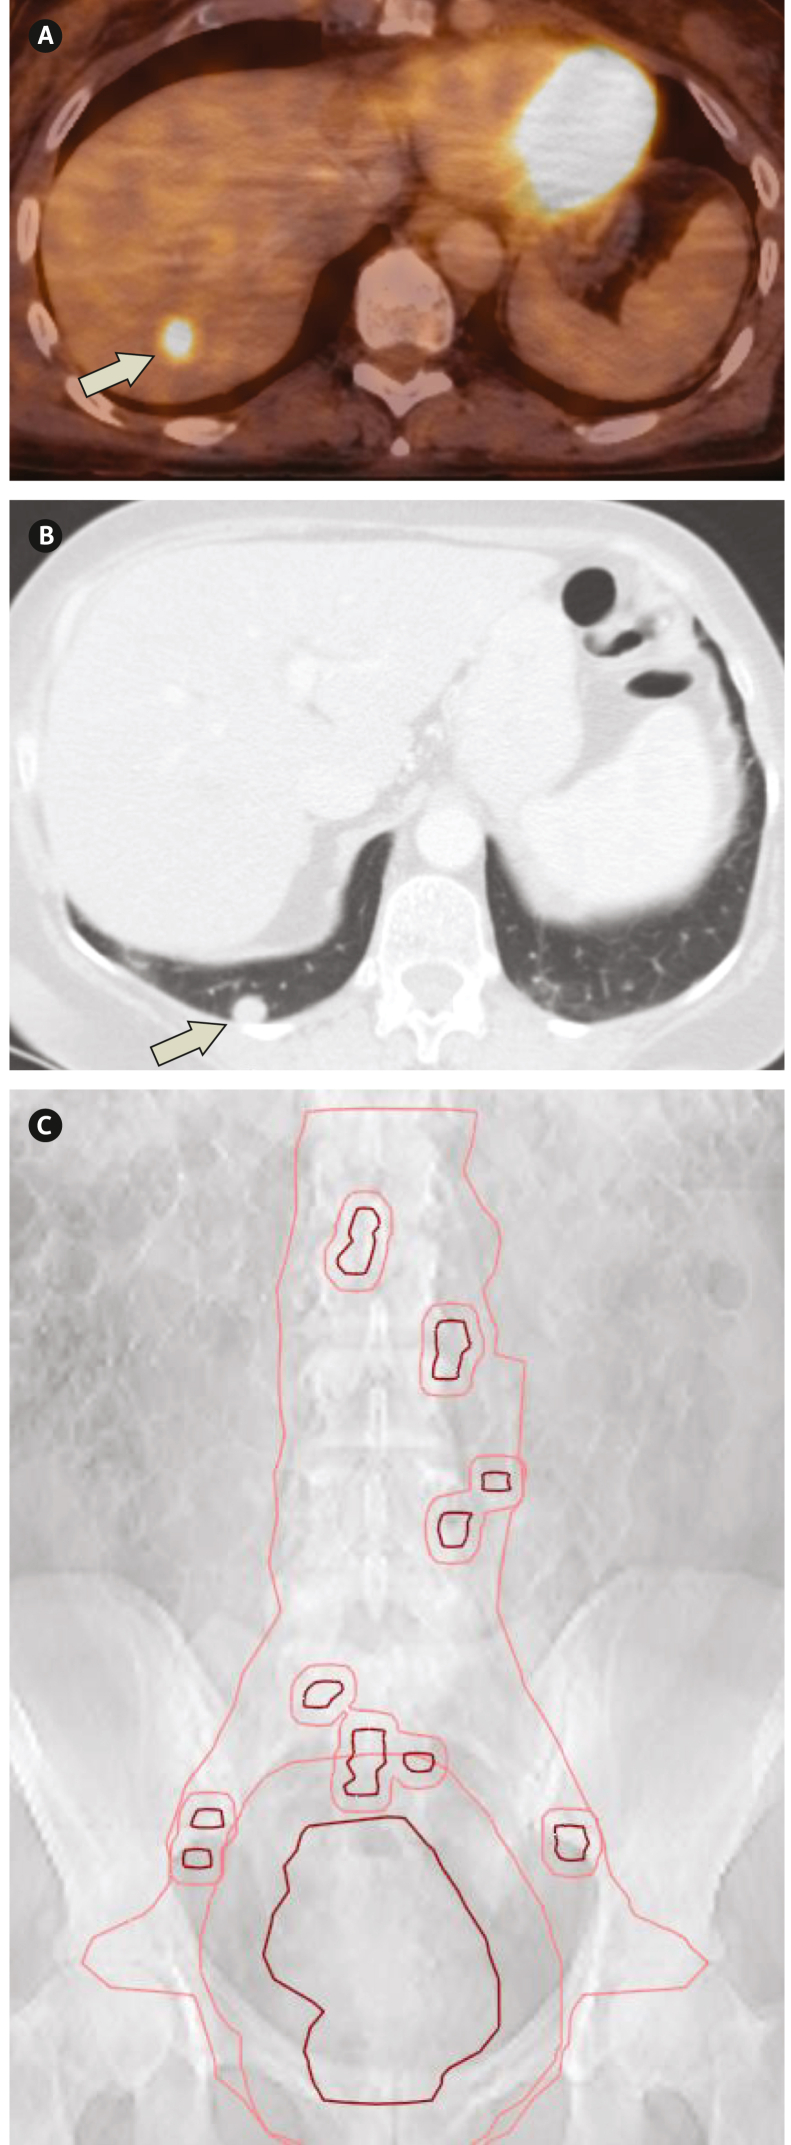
Three patients with oligometastatic anal cancer treated with curative intent. PET/CT of synchronous liver metastasis (A); CT of metachronous lung metastasis (B); topogram of radiotherapy planning gross tumor volume and clinical target volume for a patient with synchronous para-aortic lymph node metastases (C). CT, computed tomography; PET, positron emission tomography.
